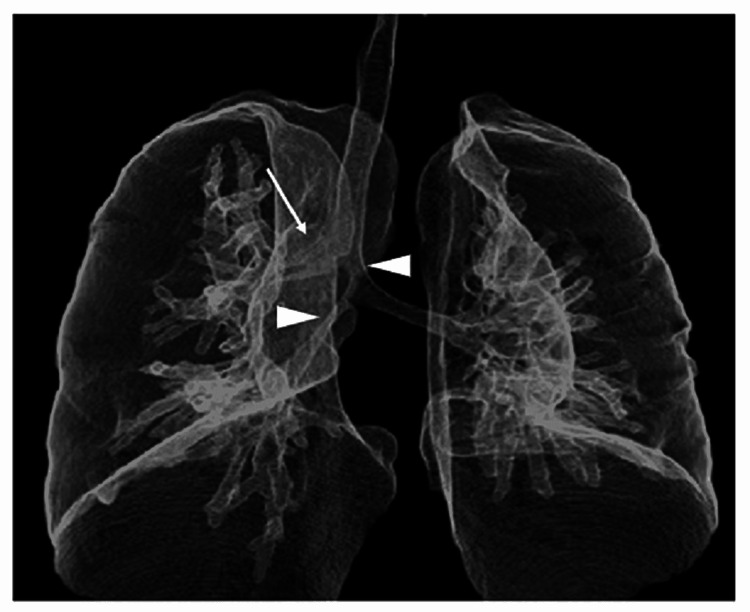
3D-CT of the chest ▷ indicates stenosis, and → indicates tracheobronchus. 3D-CT: Three-dimensional computed tomography.
